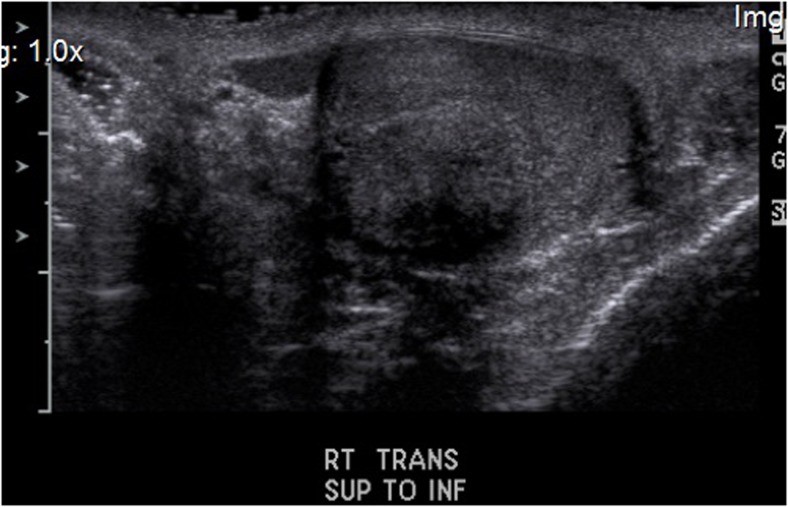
Sonographic appearance of TART
